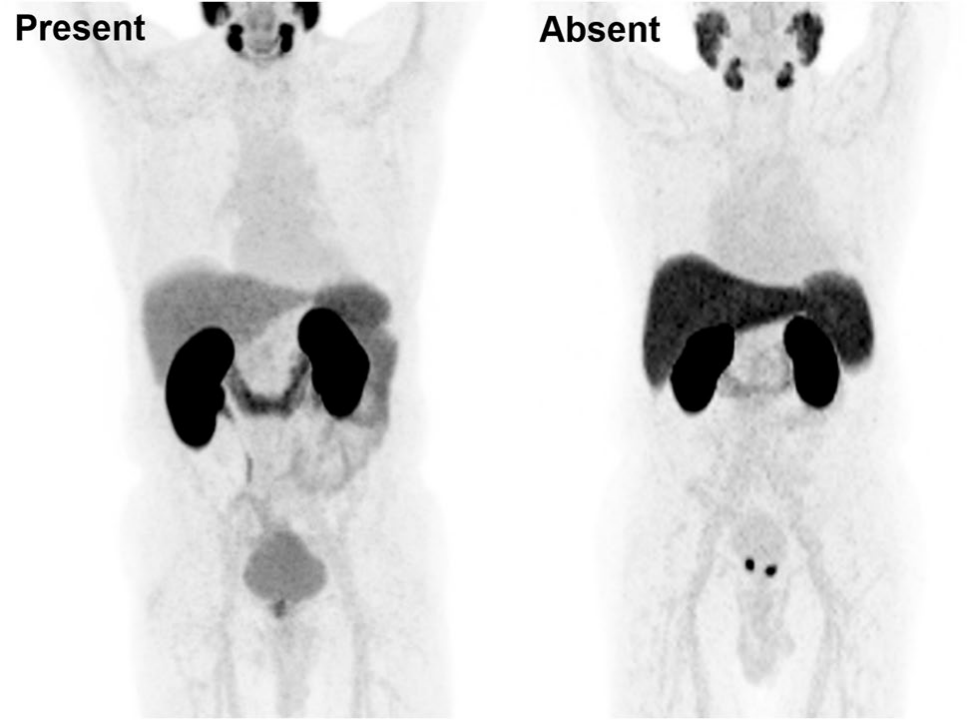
Representative qualitative assessment of the presence and absence of ureteric activity. Maximum intensity projection images captured at SUV 0–10
